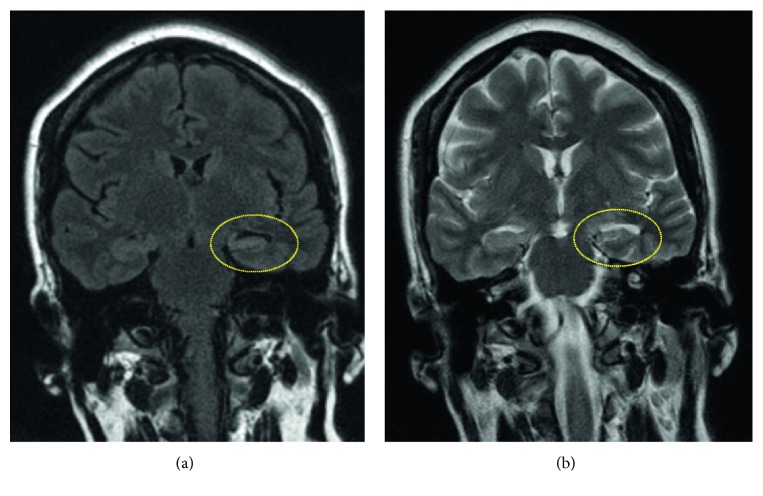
Follow-up MRI brain with contrast, 1 month after discharge, showing improved swelling of the left hippocampus with mild atrophy and volume loss on (a) FLAIR sequence coronal section and (b) T2-weighted coronal section.
